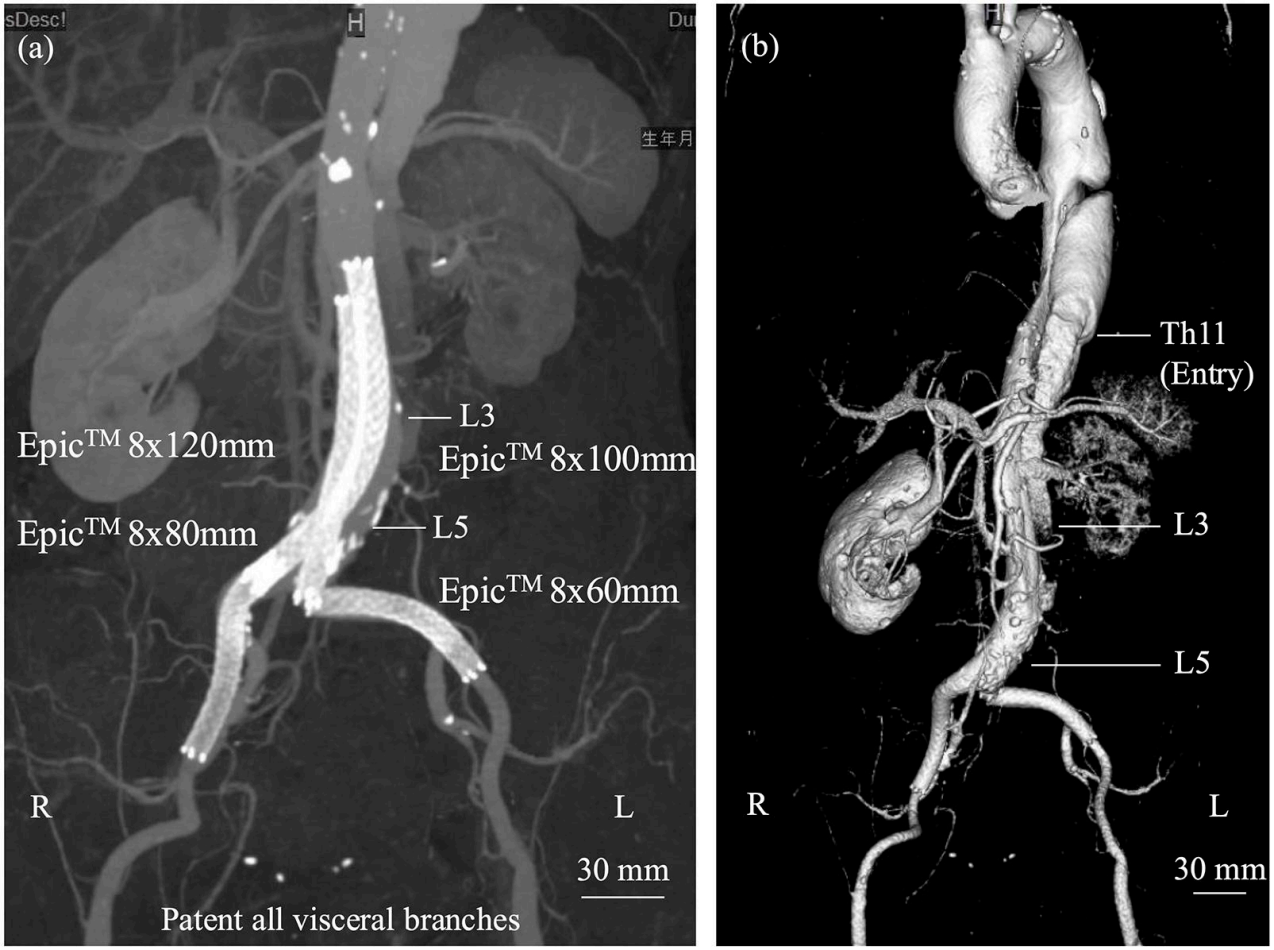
(a) Two self-expanding stents were deployed in a kissing configuration within the abdominal aorta, each extending into the respective iliac artery and relined with additional stents. (b) Post-discharge computed tomography demonstrated preservation of the true lumen from the abdominal aorta, distal to the renal arteries, to the external iliac arteries, although the left renal artery showed reduced flow without complete occlusion.
